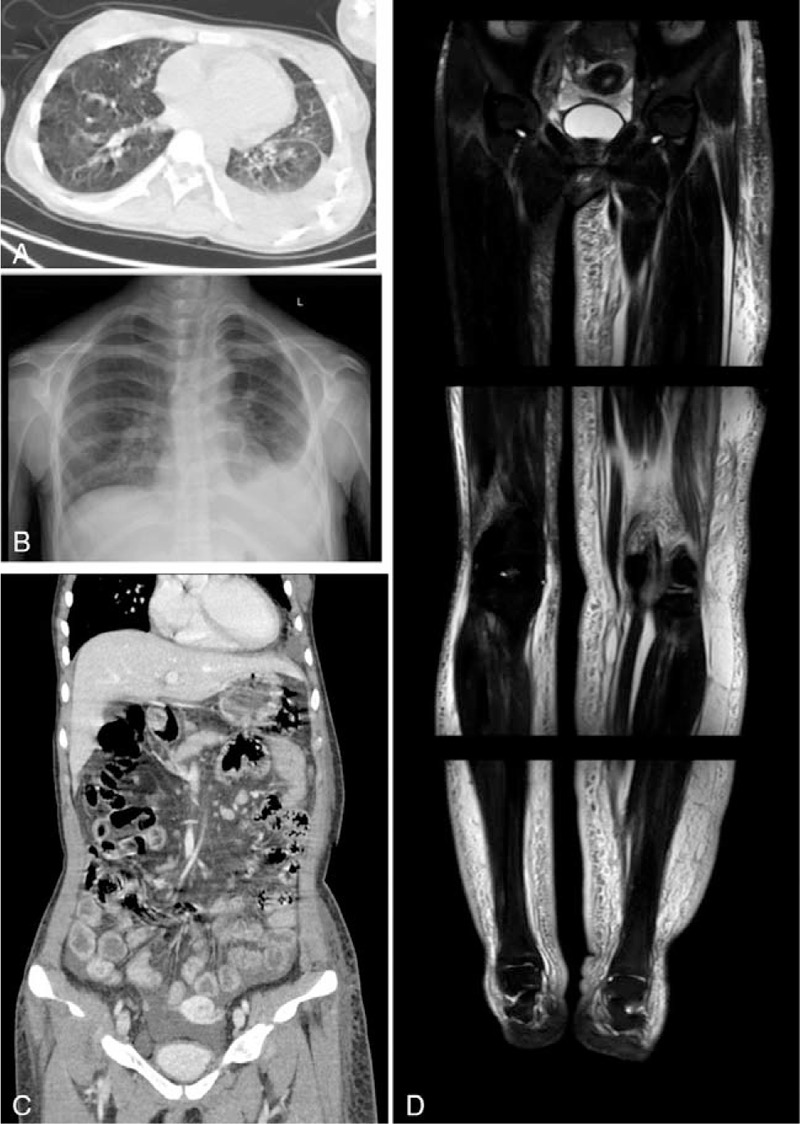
Radiographs of the proband at 20 years old. (A) and (B) Pleural effusions. (C) Thickening small intestinal wall, mesenteric edema, and minor ascites. (D) Magnetic resonance in legs show a subcutaneous deposit of lymph and fibrosis of adipose tissue.
